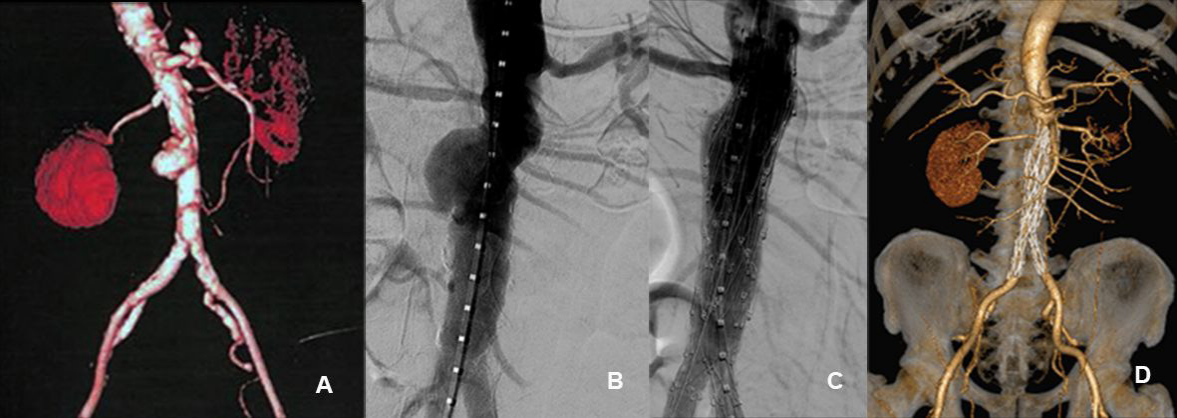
Example of saccular aortic aneurysm treated with main unibody bifurcated stent graft and proximal extension devices. (A) Pre-treatment Angio-CT image; (B) Intraoperative angiography; (C) Post-treatment angiographic appearance; (D) Post-treatment control Angio-CT.
